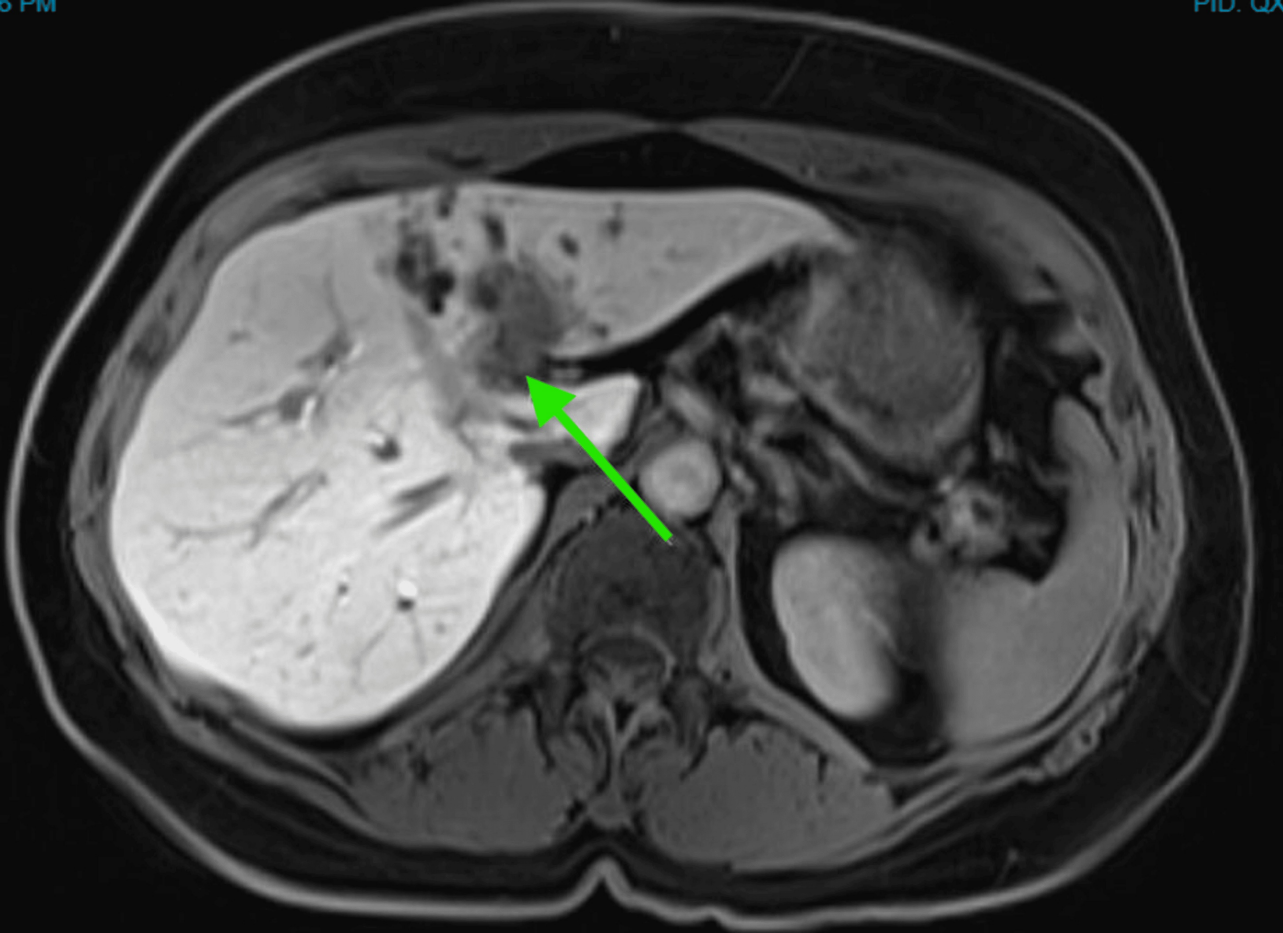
MRI demonstrated marked left lobe intrahepatic biliary dilatation with suspicious soft tissue density at the porta hepatis surrounding the left hepatic ducts Concerning for further disease was extension along the common hepatic duct and along the right hepatic duct. Green arrow: soft tissue density at the porta hepatis surrounding the left hepatic ducts
